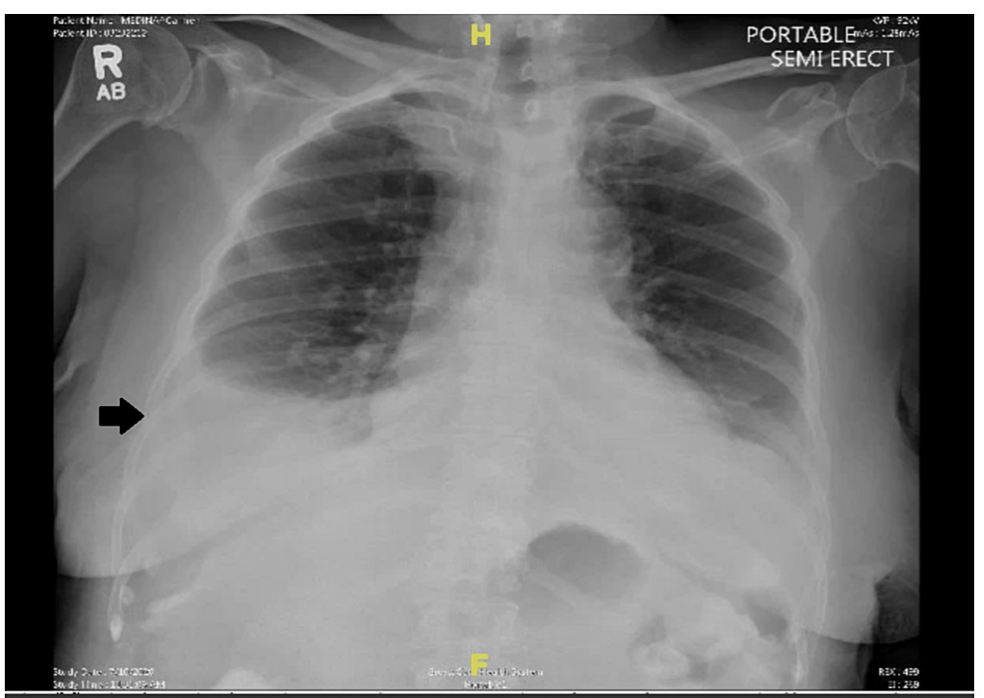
Chest radiograph showing right pleural effusion (black arrow).
